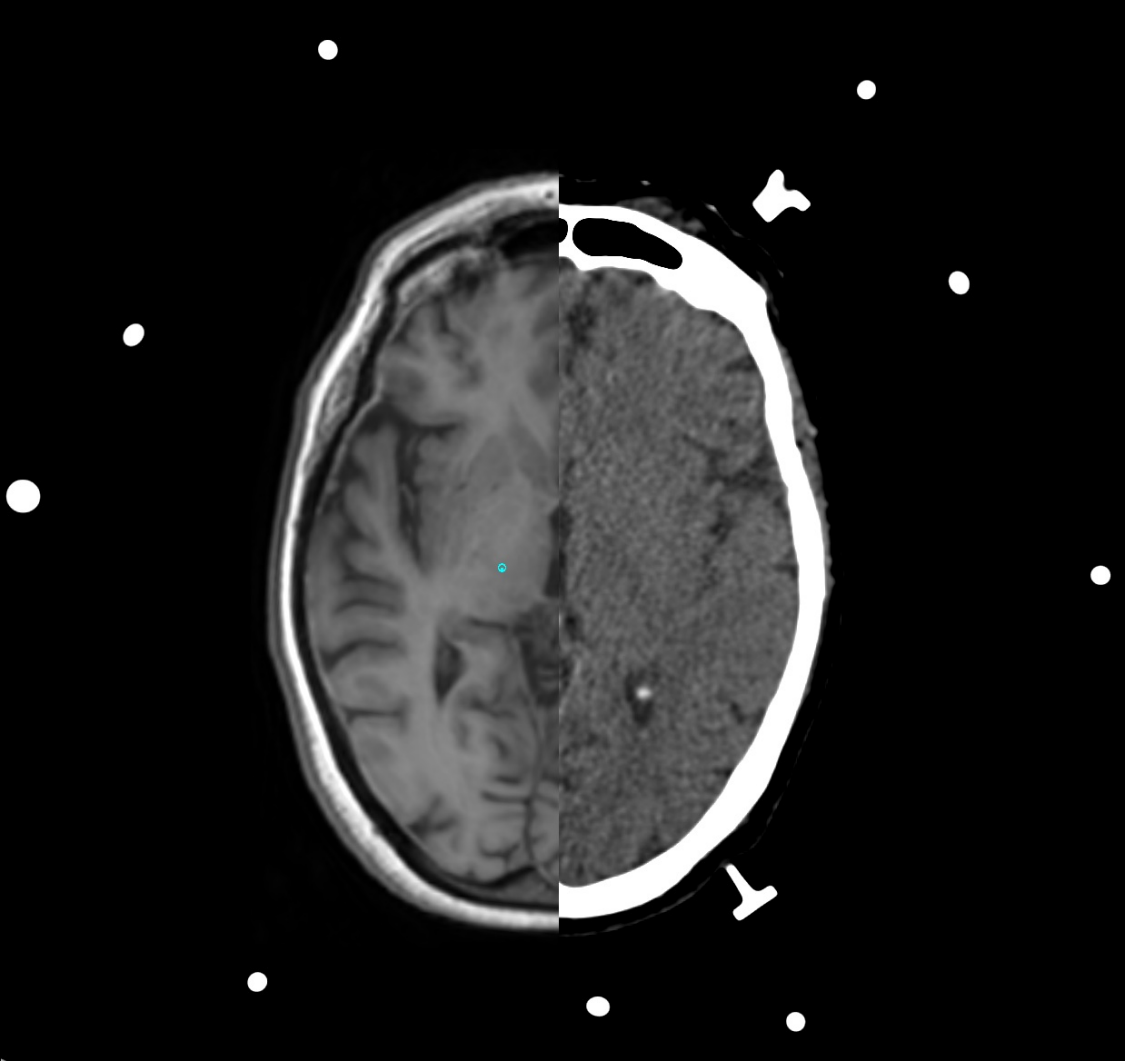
Selection of the target point A target point (depicted by the cyan circle) in the right ventro intermedius nucleus is selected from a non-stereotactic magnetic resonance (MR) image (left) that is geometrically aligned to a stereotactic computed tomography (CT) image (right). Three N-localizers create the circular and elliptical extracranial fiducials.
